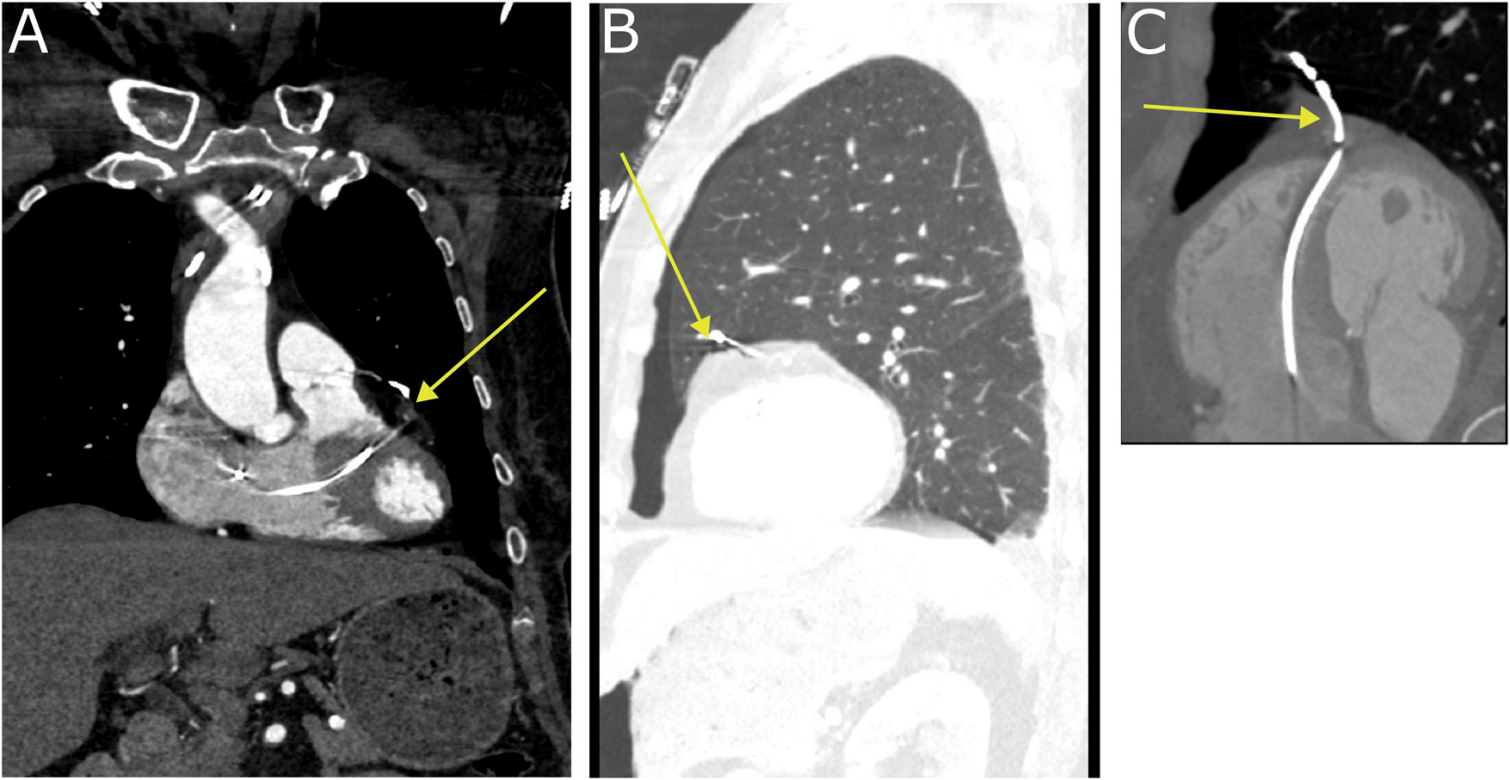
Multiplanar reconstructions from the above gated CT angiogram of the chest, showing the course of the right ventricular lead (yellow arrow) in coronal plane (A), sagittal plane (B), and along the plane of the interventricular septum (C).
